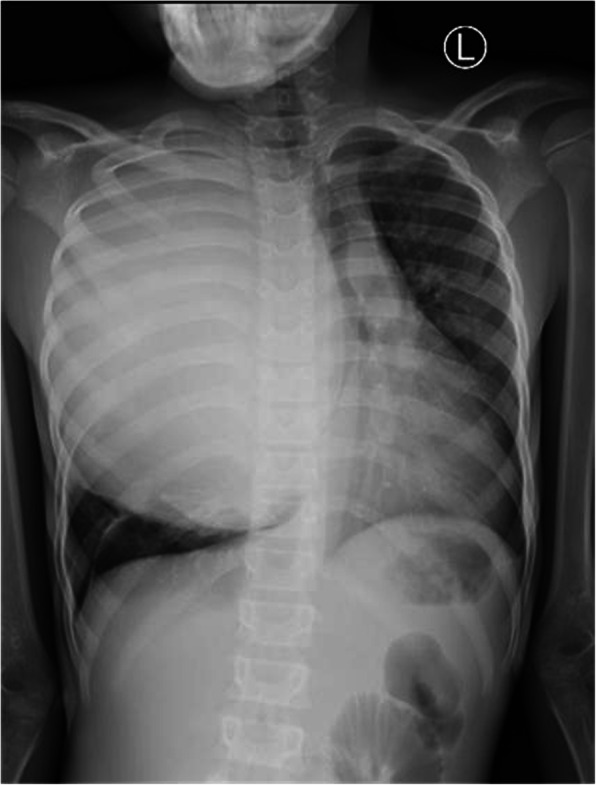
Chest x-ray posteroanterior view shows a large, rounded opacity in the right hemithorax causing mediastinal shift toward the contralateral side. Left-sided tracheal shift is seen with right main bronchus compression. No rib destruction is visualized. Features are suggestive of a hydatid cyst
